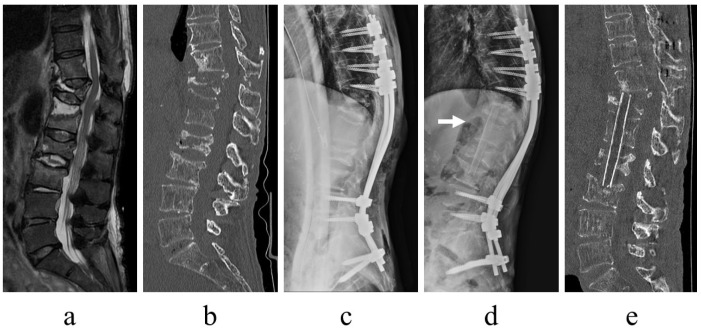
Images of a representative case with additional anterior surgery. An 81-year-old woman, T11/12/L1, L2/3 pyogenic spondylitis. Fat-saturated T2-weighted magnetic resonance imaging revealed signal changes from T11 to L3 vertebrae and disk (a), and computed tomography (CT) demonstrated large bone destruction at T12–L2, including preexisting vertebral fractures ((b), sagittal view). The patient experienced persistent back pain and underwent posterior fixation of T8–S2-alar-iliac ((c), plain radiograph of thoracolumbar lateral view). The infection persisted postoperatively, and an anterior debridement and autogenous bone grafting of the fibula were additionally performed ((d), plain radiograph of thoracolumbar lateral view, arrow, fibula; (e), CT sagittal view). Following additional anterior surgery, the infection was quickly controlled.
